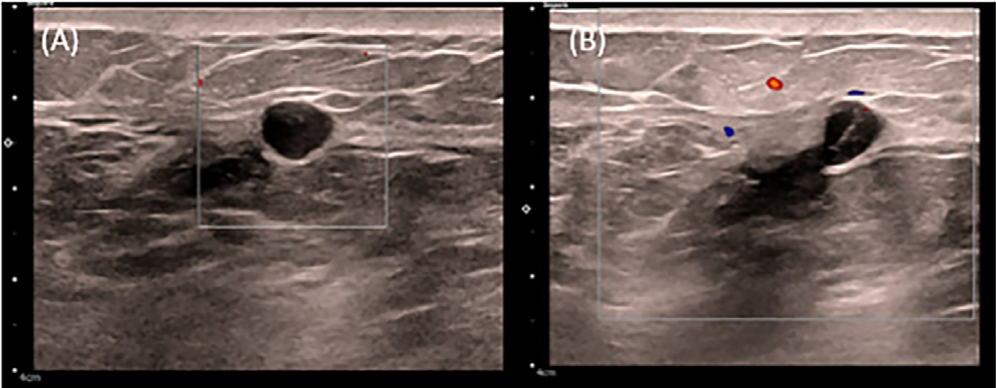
(A) Right breast targeted sonogram with color doppler shows a round, circumscribed, hypoechoic mass at 3:00 axis, 7 cm from the nipple measuring 0.7 × 0.6 × 0.8 cm. (B) Continuous with this finding, there is an elongated, irregular, hypoechoic mass measuring 1.4 × 0.6 × 1.4 cm.
